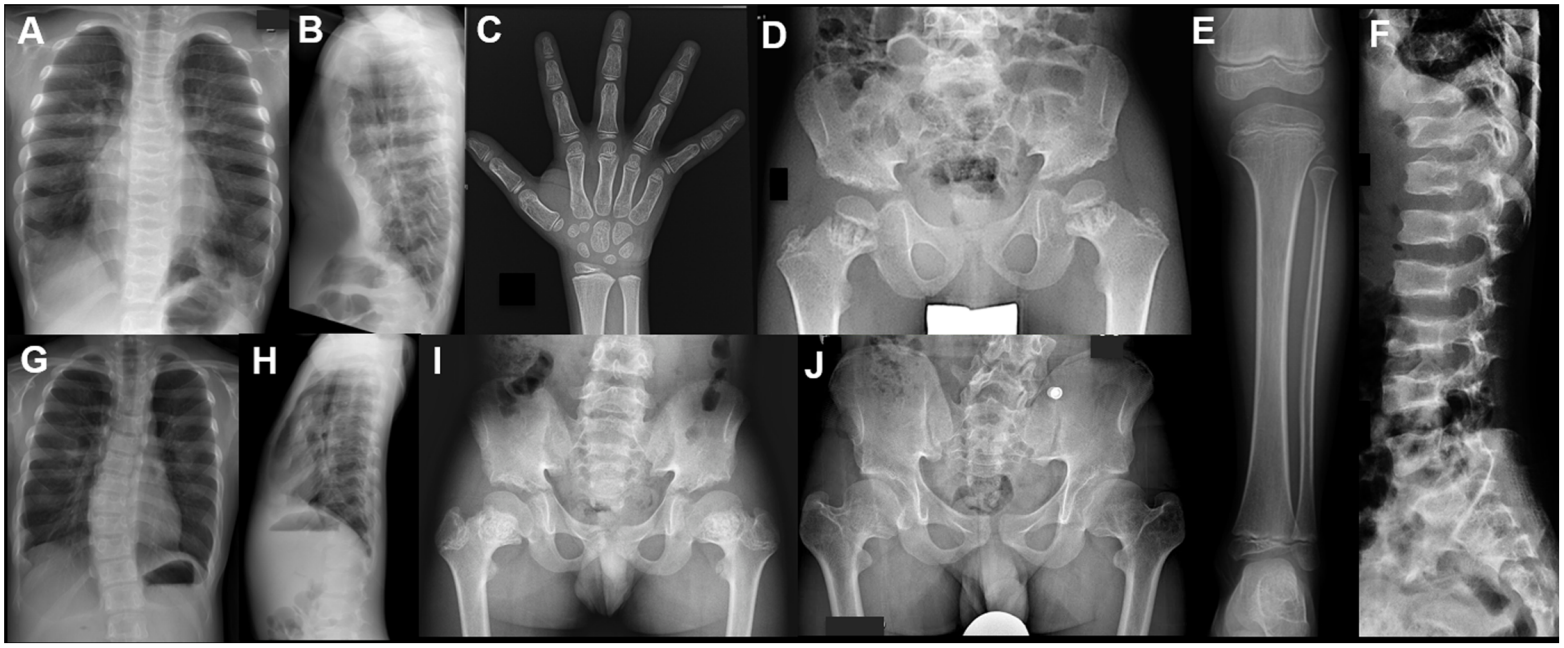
Radiographic features of axial SMD. (A-F) P7 at age 6 years. Note narrow thorax, short ribs with cupped anterior ends, mildly serrated iliac wings, short ilia, metaphyseal irregularities and shortening of the proximal femora, and mild platyspondyly. Metaphyses of knee and ankle are normal. Hands are normal. G-I) P5 at age 10 years. Narrow thorax with short ribs, mildly serrated iliac wings, short ilia, and metaphyseal irregularities and shortening of the proximal femora. He had mild scoliosis, but platyspondyly is not evident. J) P5 at age 14 years. Note progressive shortening and varus deformity of the proximal femora.
